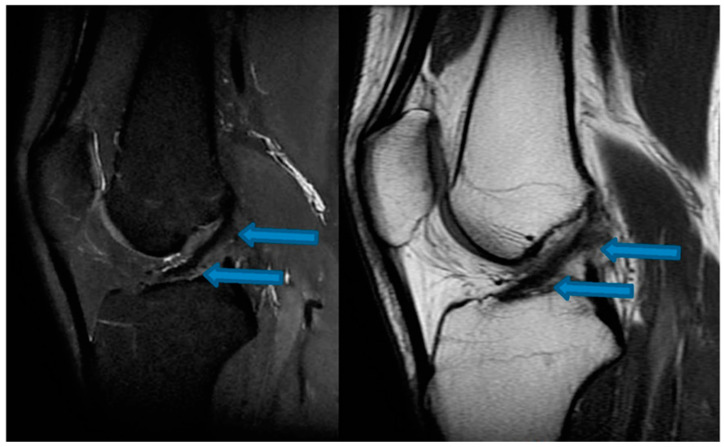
Sagittal T1- and T2-weighted MRI of the knee confirming complete healing of the ACL indicated by blue arrows (24 December 2021).
